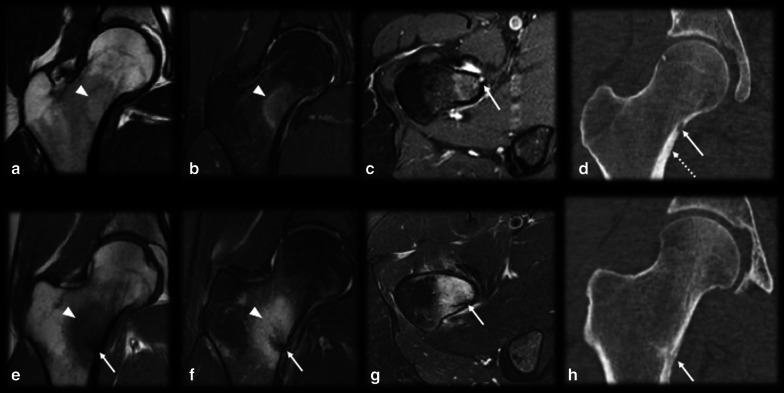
Osteoid osteoma versus calcar femorale stress fracture. (a–d) Male, 35 years old, presenting with hip pain for 3 months. MRI T1 (a) and T2 FS (b) showed bone marrow edema on the femoral neck (arrowhead), T1 FS GD (c) and CT (d) showed a small nonmineralized nidus (arrows) with gadolinium enhancement (c) and mild cortical thickening (dotted arrow in d). (e–h) Male, 33-year-old runner, presenting with hip pain for 2 weeks, which worsened during running workouts. MRI T1 (e) and T2 FS (f, g) showed bone marrow edema on the lower femoral neck (arrowhead) and a cortical fracture line (arrows), which was also seen on the CT scan (h)
